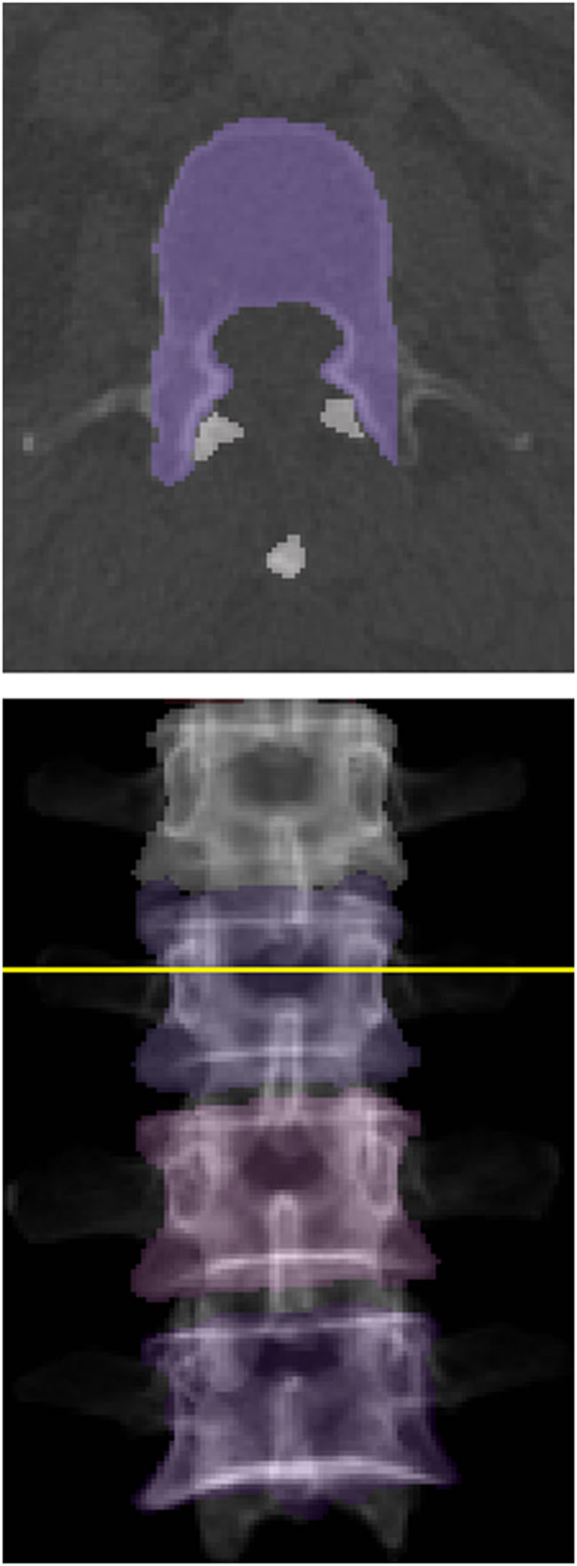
Contour of vertebra L2 in axial cut (top) used for generation of CT-based DXA of L1 to L4 in virtual posterior-anterior projection (bottom)
